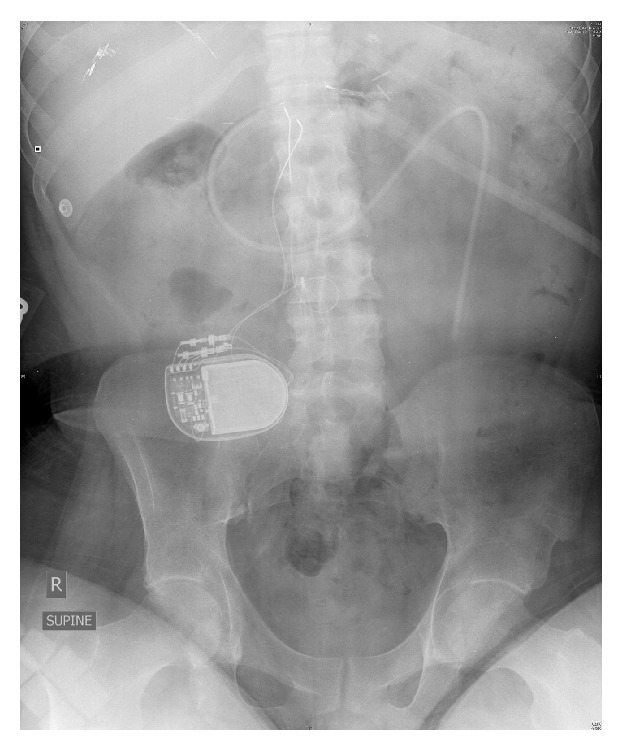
Supine radiograph demonstrating G-J tube in normal position.
